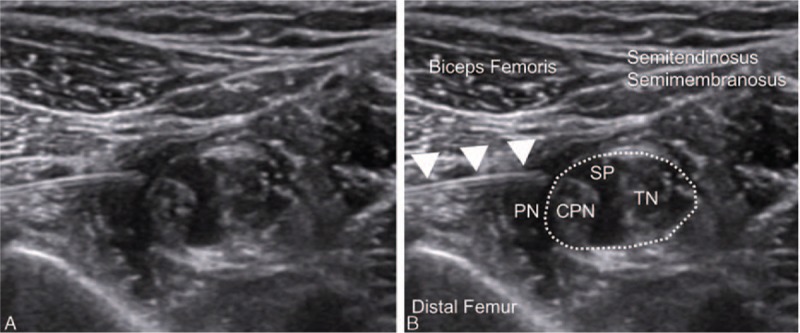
Ultrasound image of the perineural and subparaneural spaces. Shown are the paraneural sheath surrounding the sciatic nerve and the perineural space between the epimysium of the adjacent muscles and the paraneural sheath. The subparaneural space is located inside the paraneural sheath (dotted line). CPN = common peroneal nerve, PN = perineural space, SP = subparaneural space, TN = tibial nerve.
